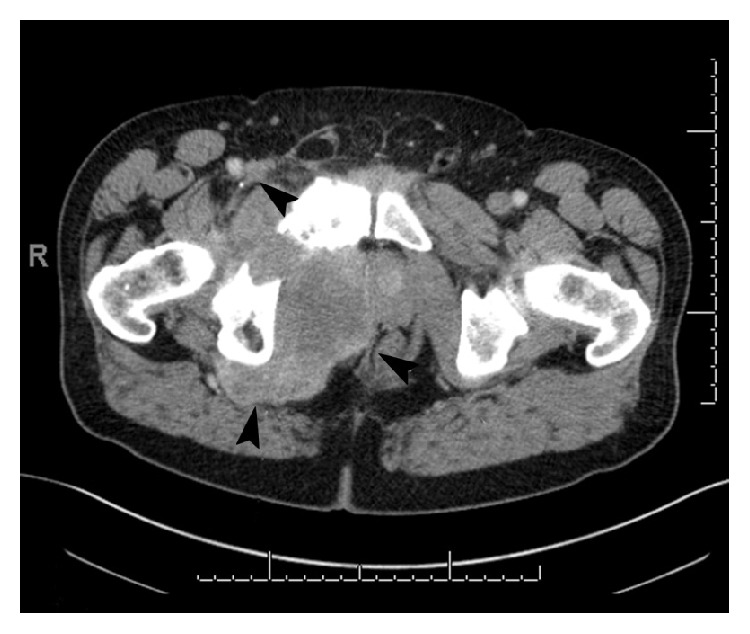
Axial CT of the pelvis (February 2015) showed severe interval enlargement of the soft tissue mass (arrows) surrounding the right inferior ramus (soft tissue algorithm).
